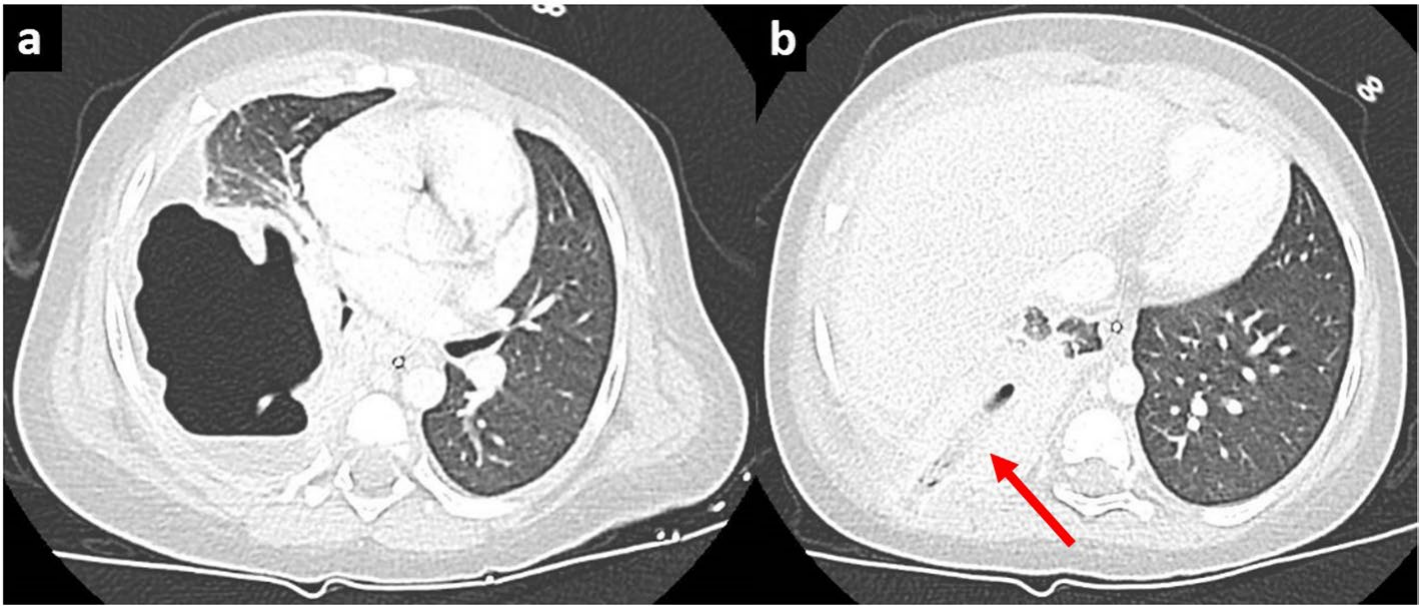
CT-scan at day 12 shows a voluminous right sub pleural empyema with an aerial component (1a), responsible for the collapse of the right lower lobe, and complicated by a pneumopleurocutaneous fistula to the right paravertebral muscles (red arrow, 1b)
